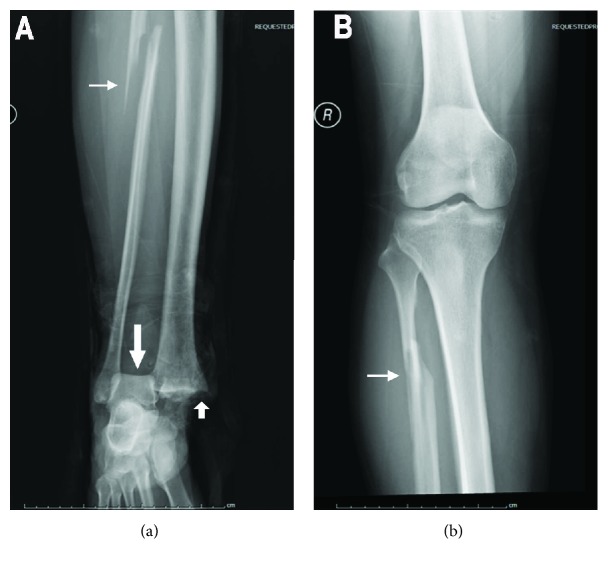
Anteroposterior radiographs of the right shin and foot and the knee joint. (a) Anteroposterior radiograph of the right shin and foot. Talus “wedged” into the ankle syndesmosis (long thick white arrow), fracture of the medial malleolus (short thick white arrow), and proximal fibular fracture (thin white arrow) are seen. (b) Anteroposterior radiograph of the right knee joint. Proximal fibular fracture is indicated with a thin white arrow.
